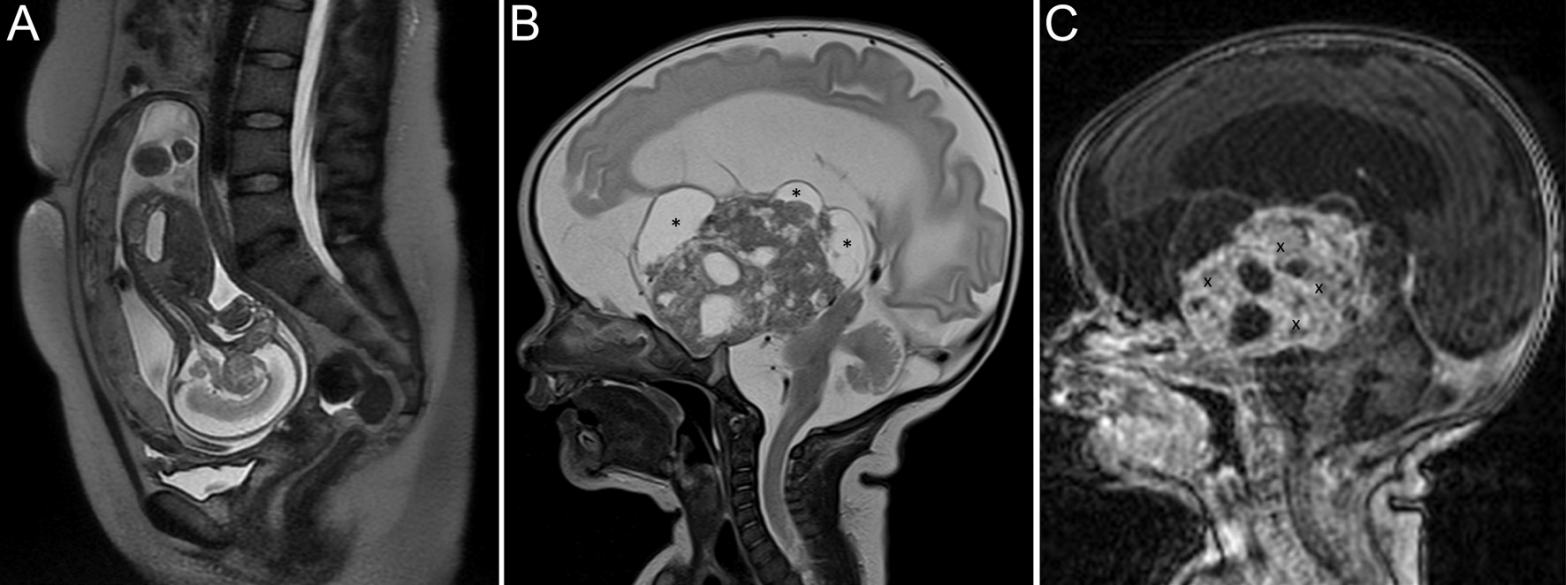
MRI of a patient with perinatally diagnosed congenital craniopharyngioma (case 3). Intrauterine MRI made at 16 weeks of gestation (A) with sagittal T2-weighted image (WI) showing a sagittal oblique plane of the fetus having a suprasellar mass in an early developmental stage of brainstem, cerebellum and cerebrum. MRI on the 15. day of life displaying growth of the cCP in all directions with huge cystic (B: black asterisks) and contrast-enhancing solid parts (C: black x’s). The brainstem, anterior, and posterior hypothalamus are compressed and displaced (B: sagittal T2WI and C: sagittal T1 WI with contrast enhancement).
